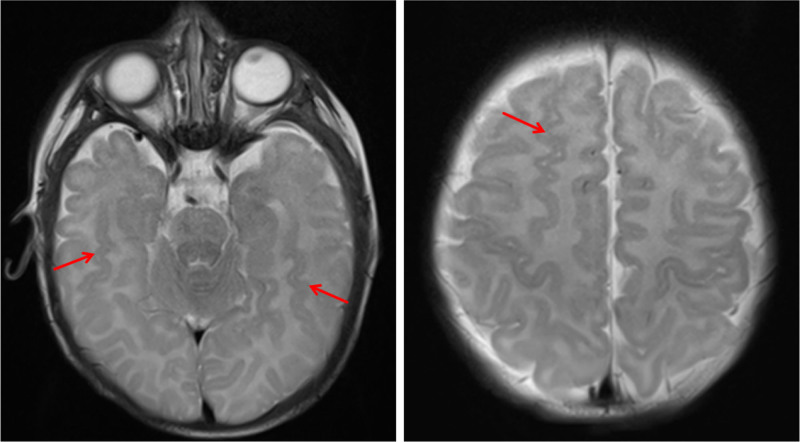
The brain MRI revealed gray matter heterotopia in the bilateral frontotemporal regions. The arrow points to the ectopic area of gray matter.
